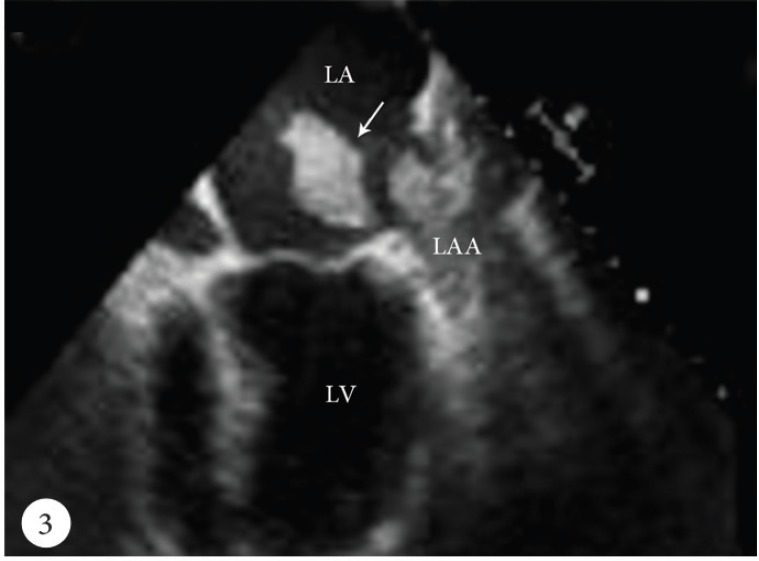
Mid-esophageal 2-chamber view of transesophageal echocardiography, showing a round mass in the left atrium and a big mass filling the left atrial appendix (arrow).
